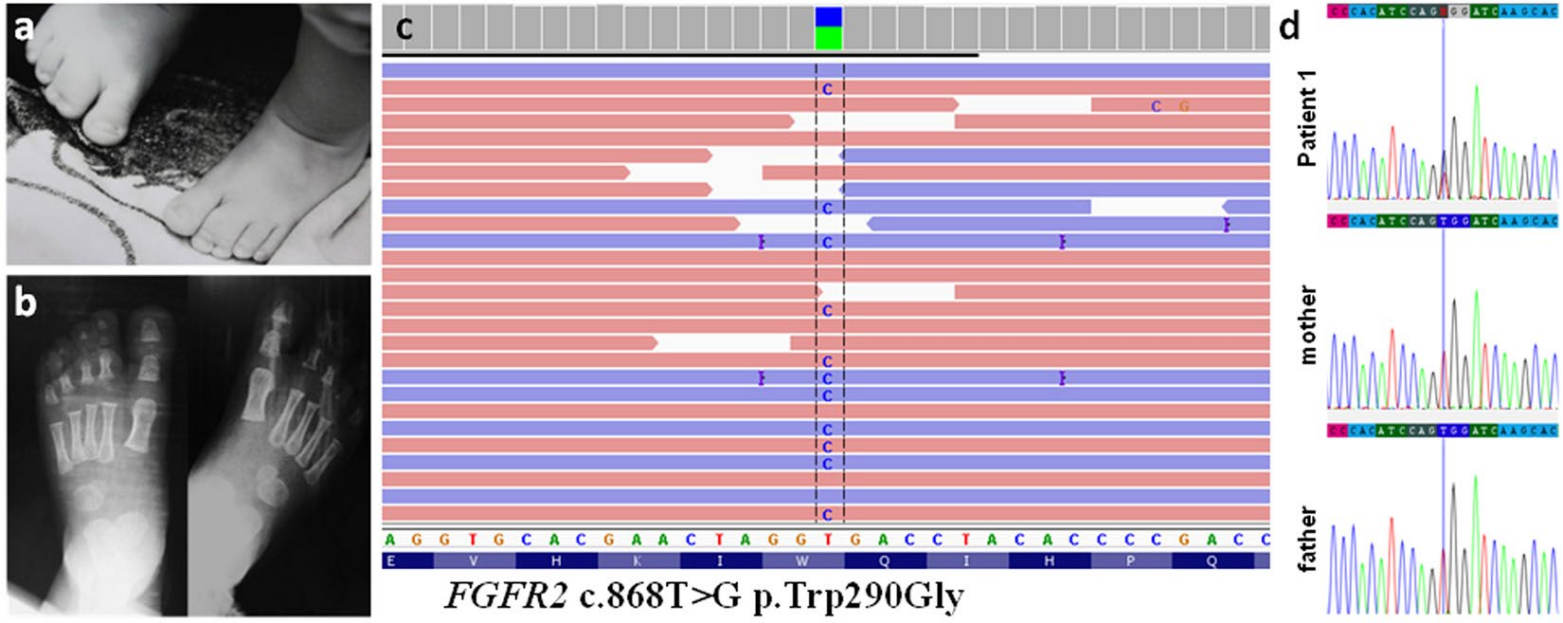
Clinical characteristics at the age of 12 months (a,b) as well as molecular results of Patient 1 (c,d). Patient 1, in addition to sagittal craniosynostosis, maxillary hypoplasia, high palate and proptosis, presented with broad halluces and skin syndactyly of 2^nd^ and 3^rd^ toes (a). X-ray of the feet showed small hypoplastic middle phalanges of all toes, relative widening of 1^st^ metatarsals and broadening of phalangeal bones forming halluces, and no bone syndactyly of 2^nd^ and 3^rd^ toes (b) Representation of the heterozygous *FGFR2* deleterious variant c.868T > G p.Trp290Gly detected in Patient 1 by means of targeted next-generation sequencing (c) and validation studies of the proband and parental testing of the *FGFR2* gene with the use of Sanger sequencing (d). Pathogenic variant c.868T > G p.Trp290Gly was confirmed in the index case and excluded in his unaffected parents, clearly indicating a de novo occurrence.
